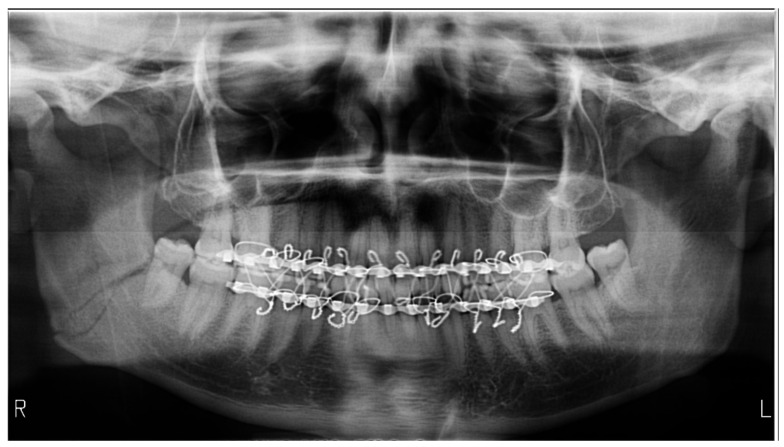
Orthopantomography of a patient with a fracture on the right angle of the mandible; bimaxillary immobilized exemplifies an improper adjustment in the focal through due to the absence of the bite-block, resulting in a blurry contour of the teeth, more obvious in the frontal region. Additionally, the image shows a head position in extension and incorrect exposure factors.
